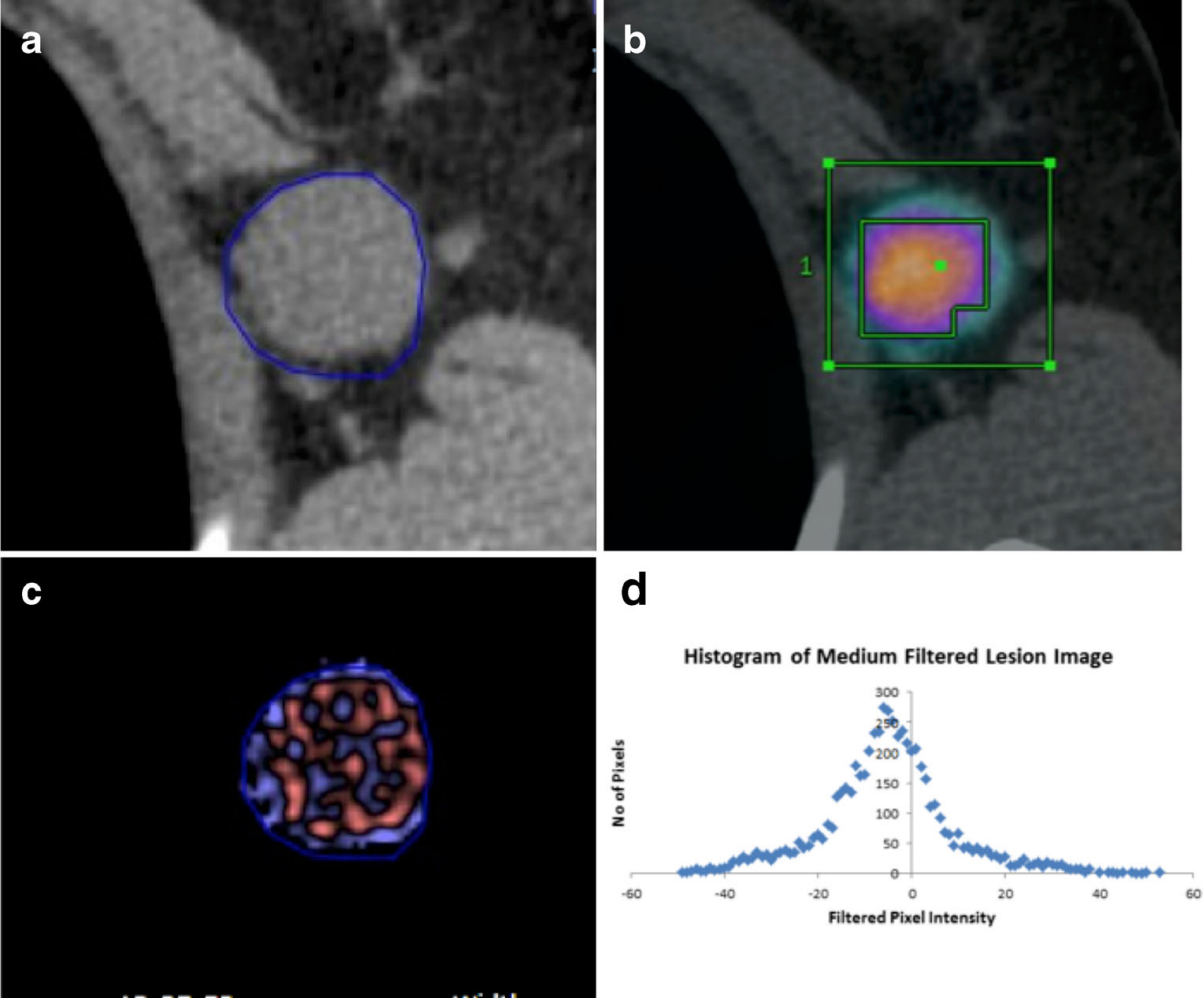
Multi-parametric PET-CT of non-Hodgkin lymphoma comprising CT image (a), fused FDG uptake on PET and CT image (b), CT texture map highlighting features at medium texture scale (c), and CT texture histogram (d)
